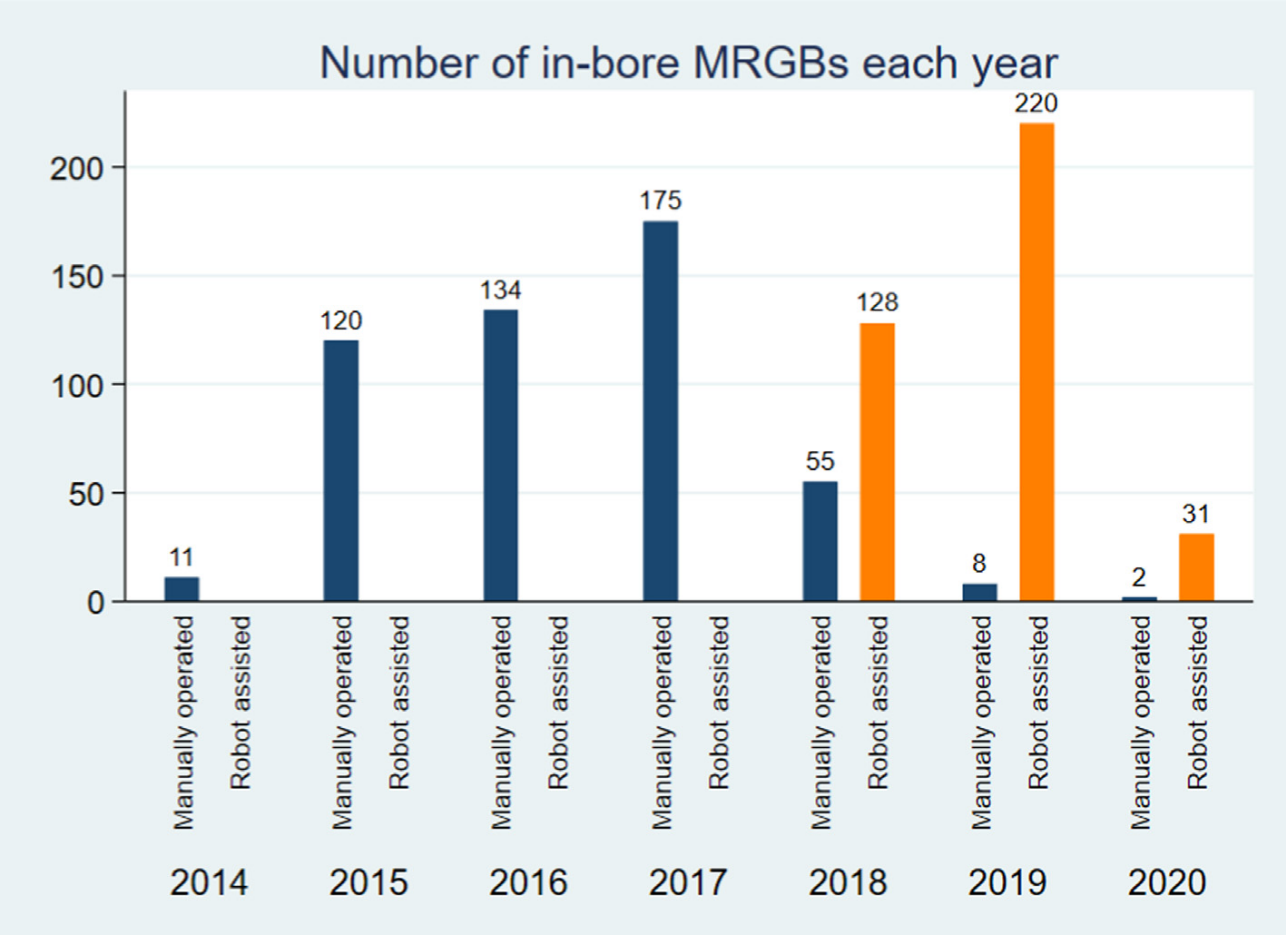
Number of in-bore magnetic resonance imaging targeted prostate biopsies (MRGBs) each year with the manually operated in-bore MRGB system and the robot-assisted in-bore MRGB system. From August 2014 through February 2018, all patients had in-bore MRGB performed with the manually operated system. From March 2018, the in-bore MRGB system of choice was the robot-assisted system and the manually operated system was used only when the robot-assisted was unavailable due to maintenance.
